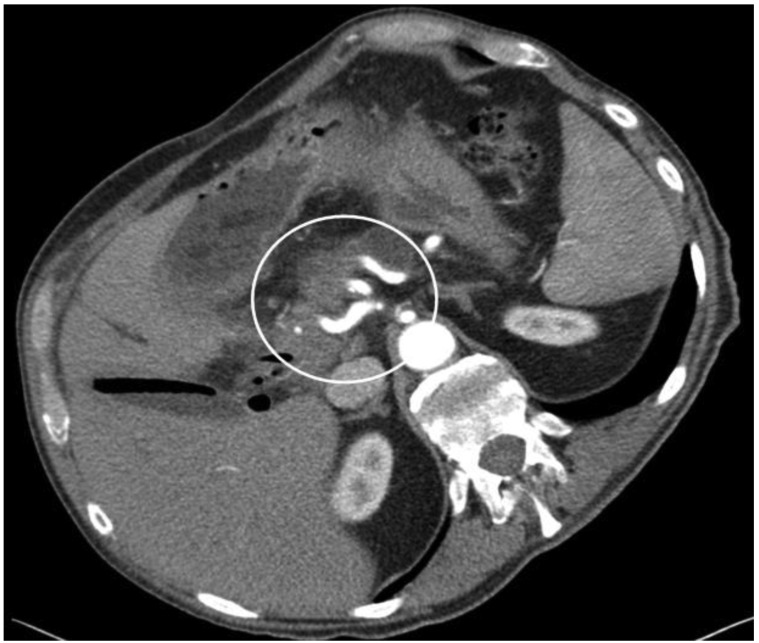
CT scan showing pancreatic head cancer with infiltration of the celiac trunk. Infiltration of common hepatic, left gastric and splenic artery (white circle, left, middle and right vessel). Technically not resectable finding.
